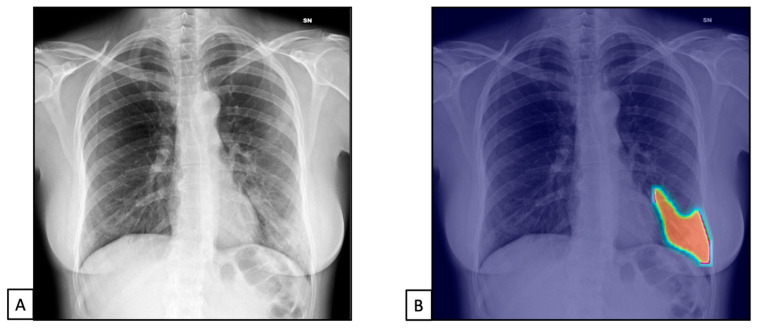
Chest X-rays of patients admitted to the emergency department with respiratory distress and fever belonged to dataset 2. (A) represents CXR acquired at the bedside, showing compact typical opacities in the left lung lower zones. (B) represents AI system analysis, showing the pathological zones. The AI system reported a high suspicion of pneumonia, not typical for COVID-19 (99.99%). The final diagnosis was lung pneumonia due to Streptococcus pneumoniae.
